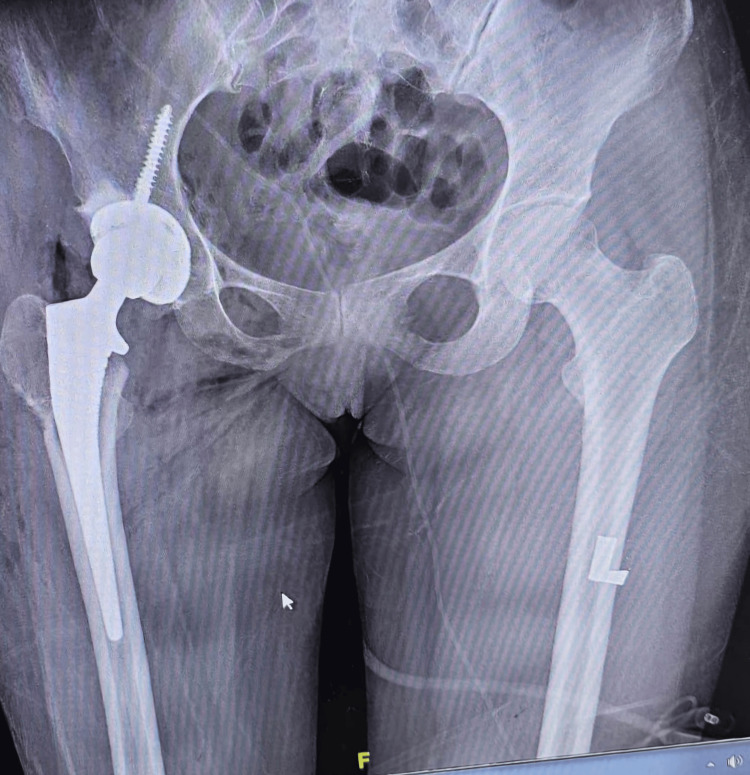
Postoperative X-ray of patient 9.
